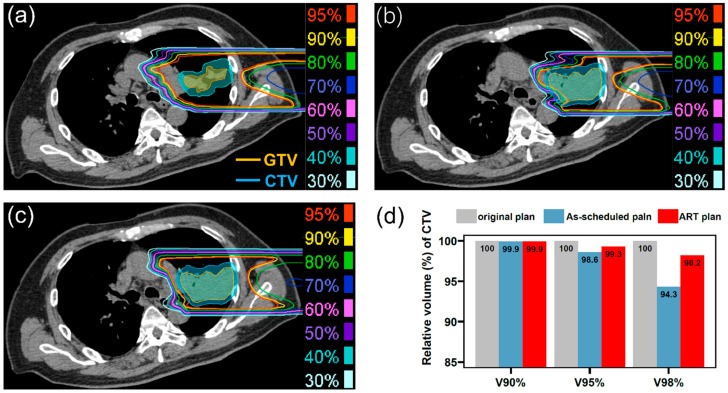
(a) Dose distribution of a field in the original treatment plan on the plan CT (4 Gy[RBE]), (b) dose distribution of the field in the as-scheduled plan on the weekly CT (4 Gy[RBE]), (c) dose distribution of adapted field on the weekly CT (4 Gy[RBE]), and (d) dose–volume histogram parameters between these plans. GTV, gross tumor volume; CTV, clinical target volume; ART, adaptive radiotherapy; RBE, relative biological effectiveness.
